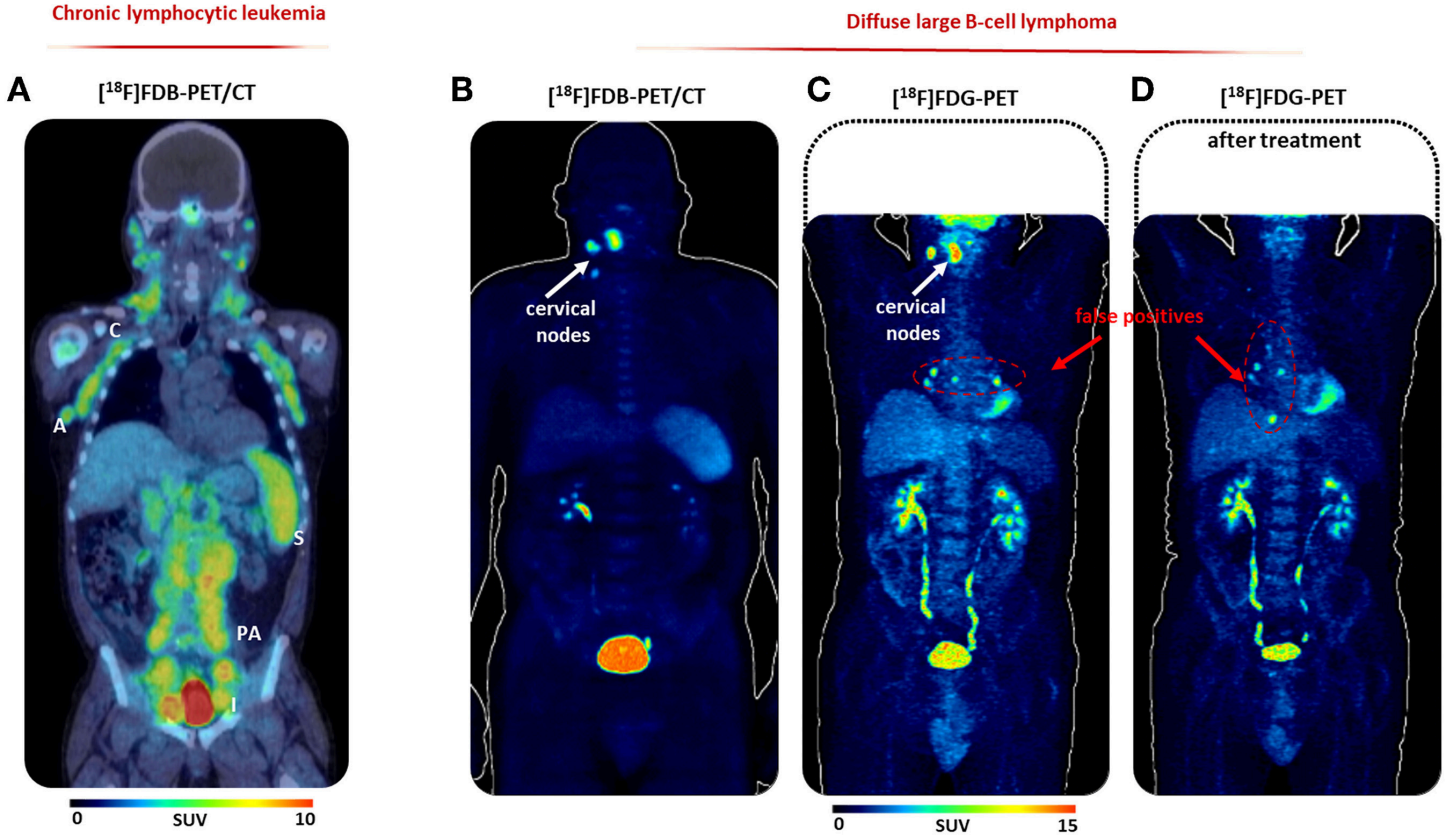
First-in-man study. (A) [^18^F]FDB-PET/CT (30–50 min scan period) of a representative CLL patient; (B) [^18^F]FDB-PET (30–50 min scan period), (C) [^18^F]FDG-PET (60–80 min), and (D) post-treatment (60–80 min) of a representative DLBCL patient, reproduced from Chantepie et al. (18), no permission required. A, axillary nodes; C, cervical nodes; I, iliac nodes; PA, paraaortic nodes; S, spleen.
